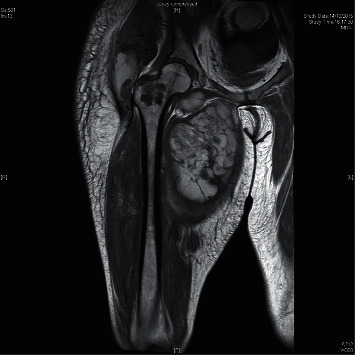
Magnetic resonance imaging (T2-weighted) of the right hip.
